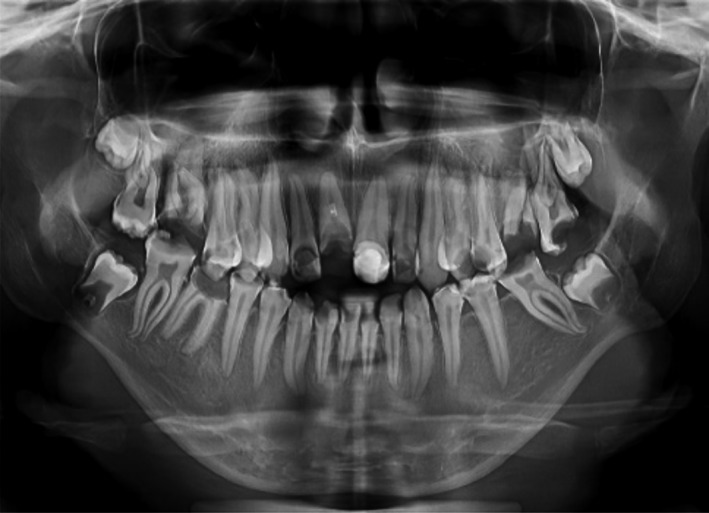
Orthopantomography of the patient.
